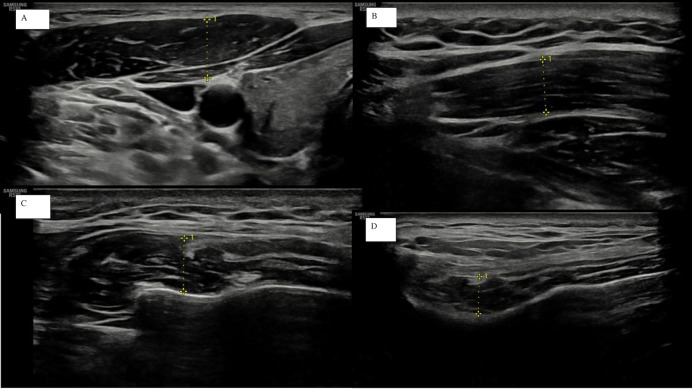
Representative ultrasonographic images of the measured muscles. (A) Sternocleidomastoid, (B) upper trapezius, (C) masseter, and (D) temporalis. Measurement lines indicate muscle thickness defined as the distance between superficial and deep fasciae.
